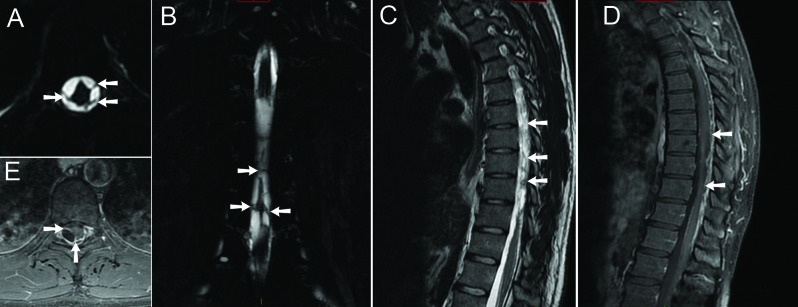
A. Axial fatsuppressed (FS) T2-weighted image (WI). B. Coronal FS T2-WI. C. Sagittal T2-WI. D. Sagittal FS T1-WI after administration of intravenous gadolinium contrast. E. Axial FS T1-WI after administration of intravenous gadolinium contrast.
